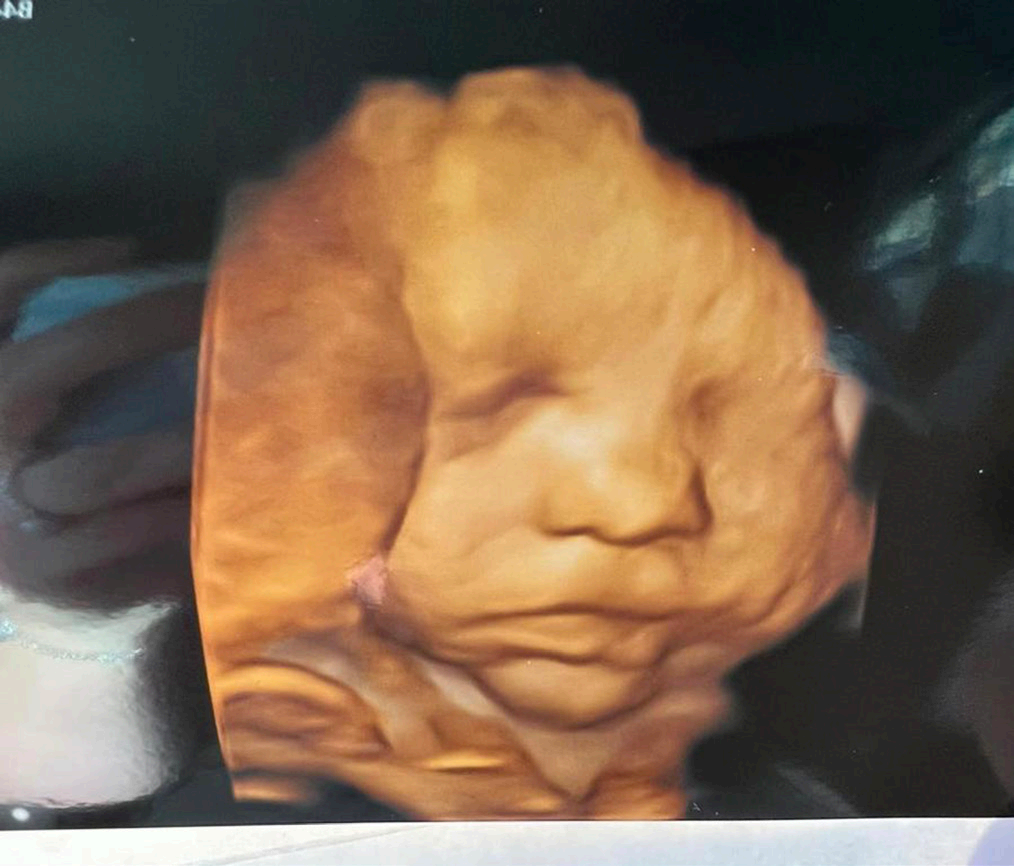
The volume rendered pre-natal ultrasound image performed about 5 weeks before delivery, in the retrospect, depicts facial asymmetry on the right side.
